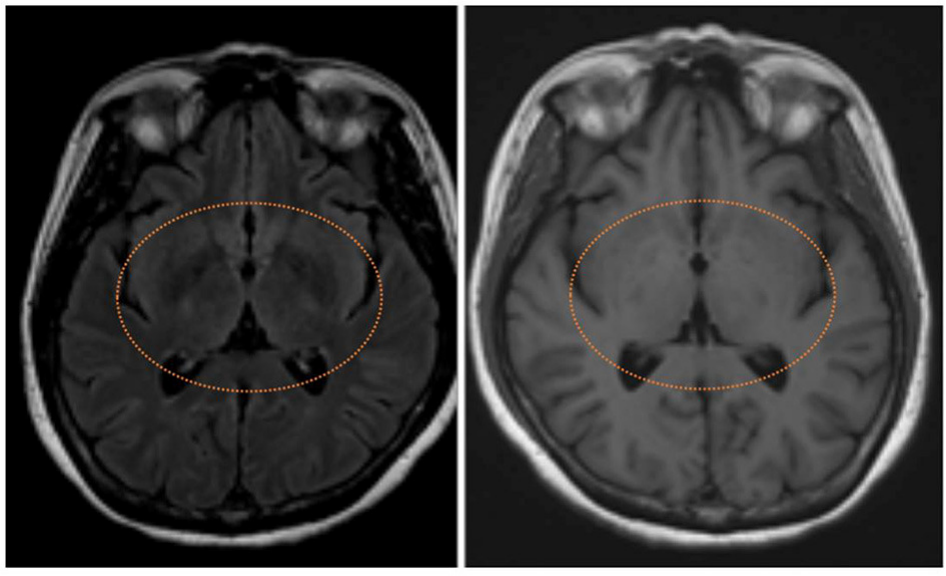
T2 fluid-attenuated inversion recovery magnetic resonance image showing symmetrical hyperintensities in bilateral basal ganglion (left) with T1 hypointensities (right) of neonicotinoids intoxication patient.
